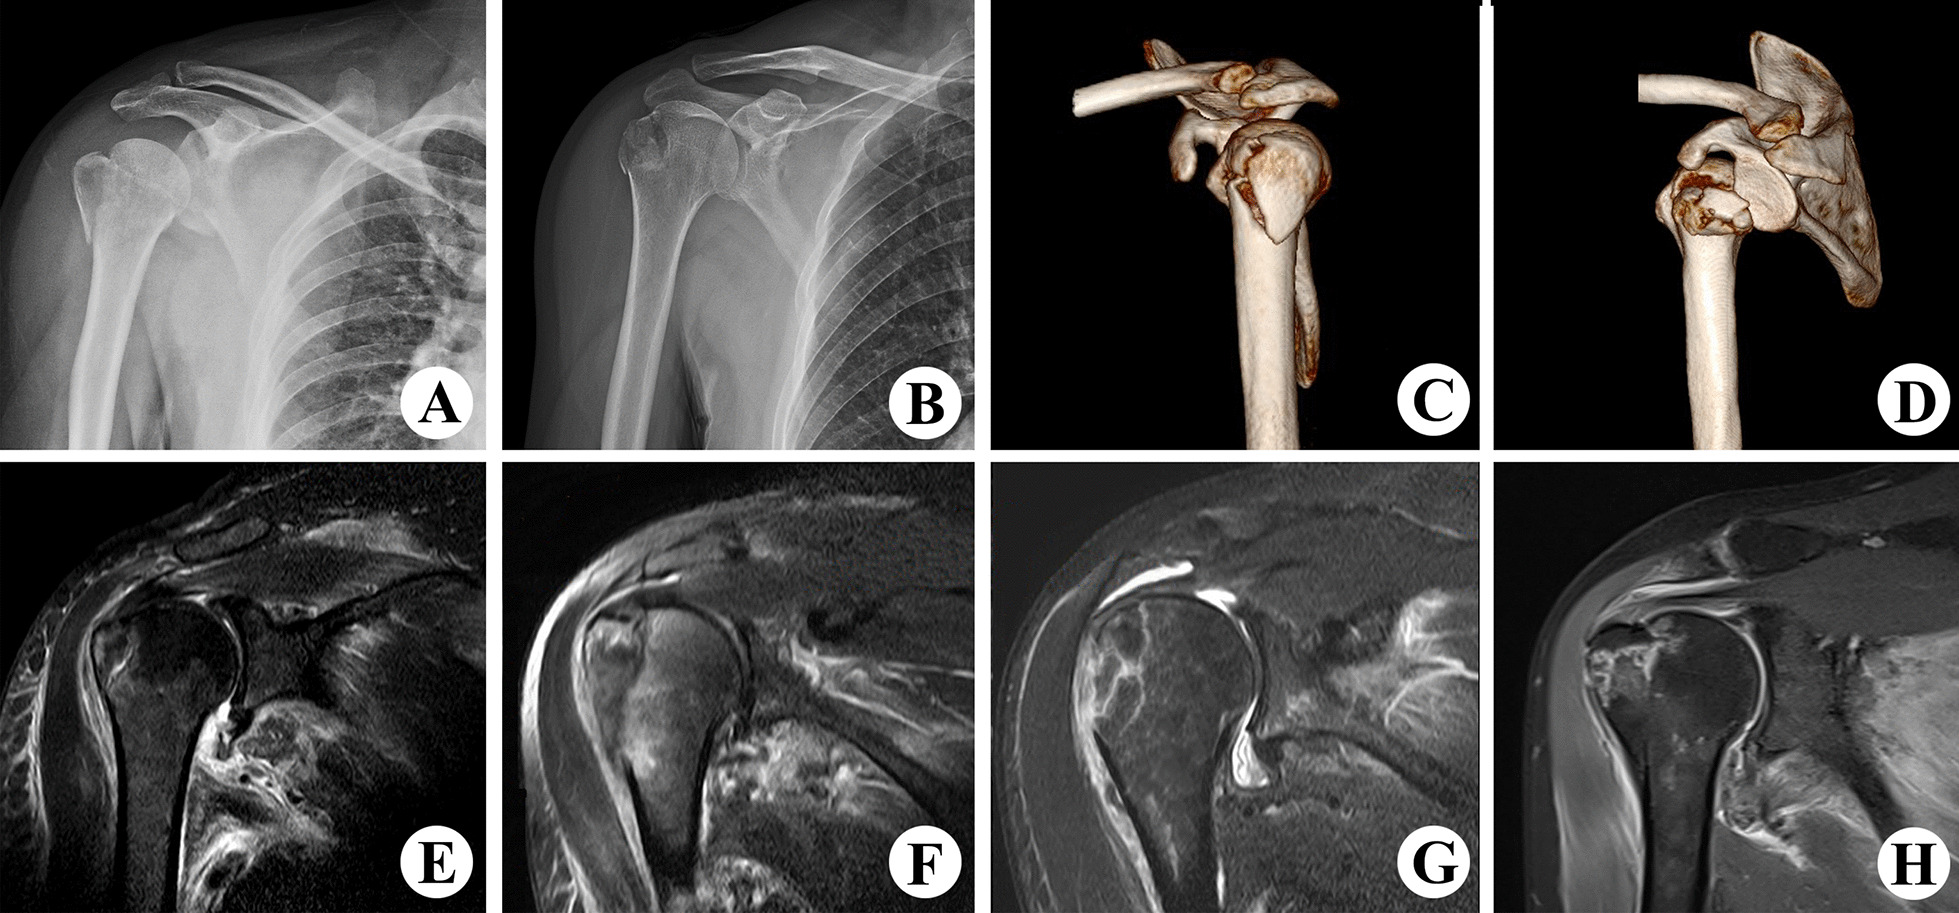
Clinical imaging findings of fractures of the humeral greater tuberosity. A X-ray image of a single-fragment fracture. B X-ray image of a multi-fragment fracture. C Computed tomography scan of a single-fragment fracture. D Computed tomography scan of a multi-fragment fracture. E Magnetic resonance image of a single-fragment fracture. F Magnetic resonance image of a single-fragment fracture with rotator cuff tear. G Magnetic resonance image of a multi-fragment fracture without rotator cuff tear. H Magnetic resonance image of a multi-fragment fracture with rotator cuff tear
